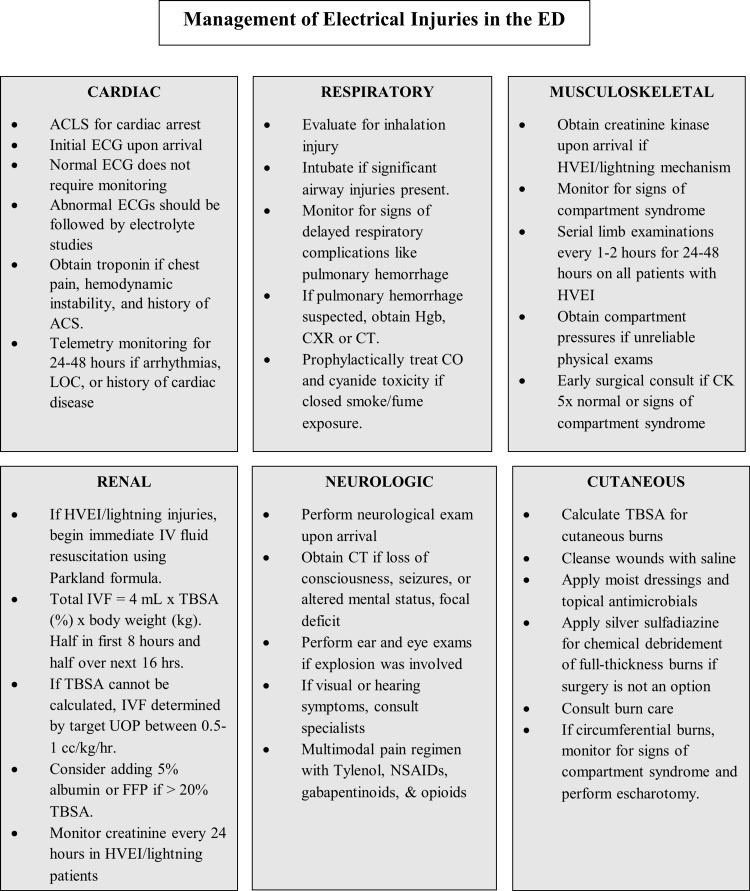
Management of electrical injuries by organ system in the ED. Figure Credits: Ireland Smith, Savannah Kidd ACLS: Advanced cardiac life support; ACS: Acute coronary syndrome; CK: Creatine kinase; CO: Carbon monoxide; CXR: Chest x-ray; FFP: Fresh frozen plasma; HVEI: High-voltage electrical injury; IVF: Intravenous fluids; LOC: Loss of consciousness; LVEI: Low-voltage electrical injury; NSAIDs: Nonsteroidal anti-inflammatory drugs; TBSA: Total body surface area; UOP: Urine output.
